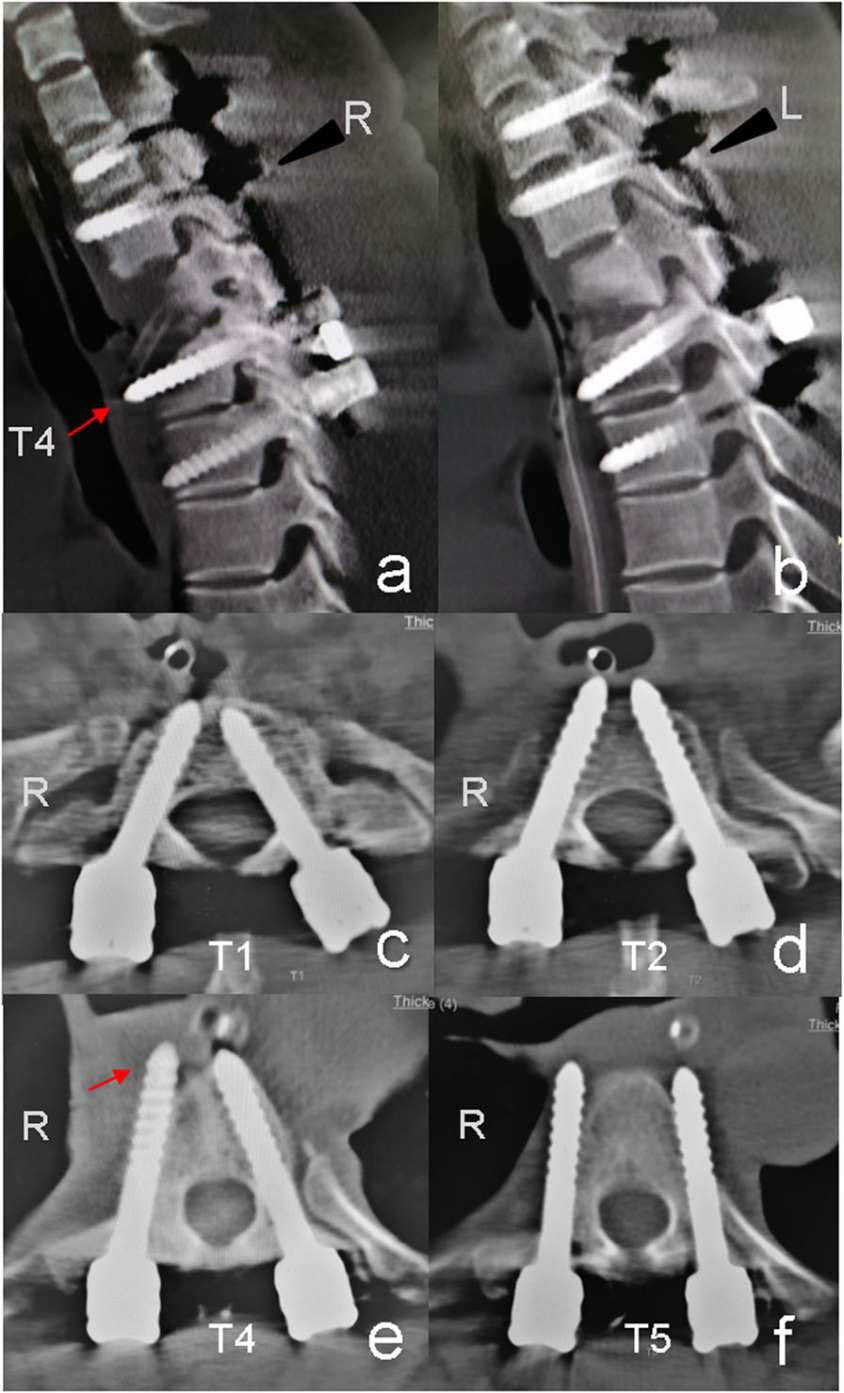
CT examination showed that the right screw of T4 vertebra was too long, penetrated the anterior edge of vertebra and occupied the anatomical position of oesophagus (red arrow), sagittal cuts along with direction of the screws were showed in a-b and axial cuts at all levels were showed in c-f
